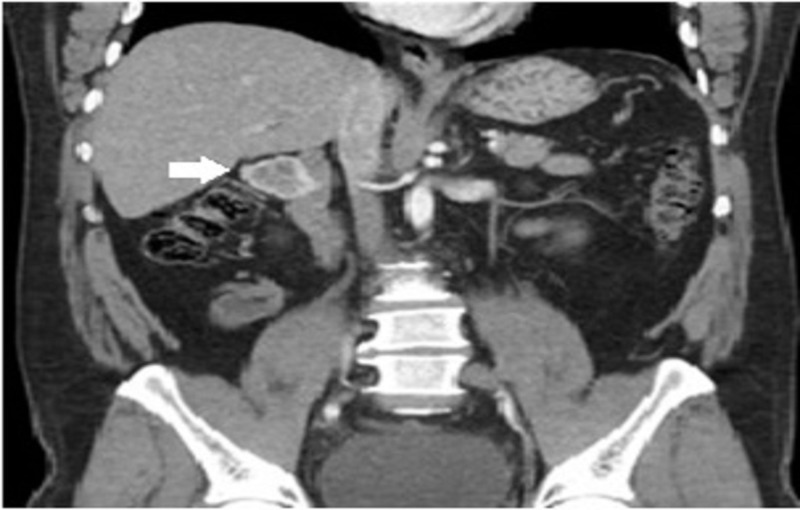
Coronal view of contrast computed tomography scan of the abdomen showing a 3.5 cm mass (white arrow) located laterally to the second part of the duodenum, anteriorly to the right kidney and posteriorly to the hepatic flexure. The mass was separate from the adrenal gland and demonstrated a heterogeneous ring enhancement and contained some coarse calcifications anteriorly.
